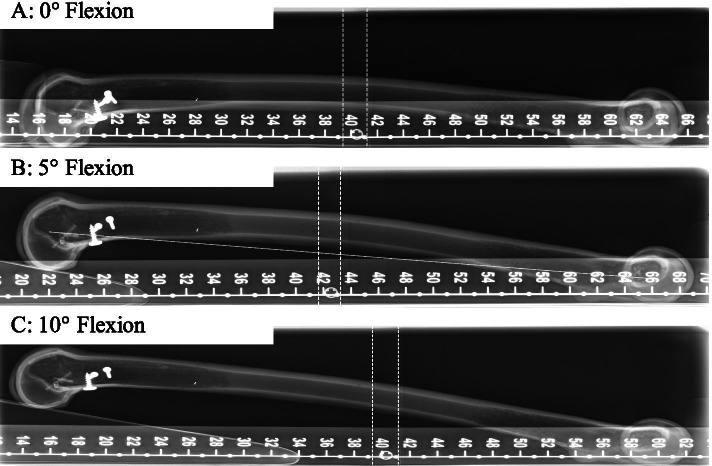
Experimental setup of radiographic imaging for flexion error. Different flexion error setups with 0° flexion error (a), 5° flexion error (b) and 10° flexion error (c). Scale bar used for angle measurement and calibration
